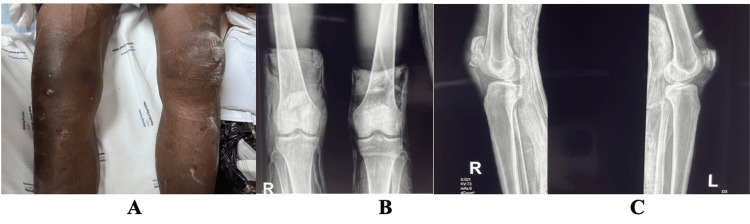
Clinical image and radiographs A: a clinical picture of the superficial abrasion on the legs. B and C: radiographs of the bilateral patellae - anteroposterior and lateral views showing fracture of the patellae
